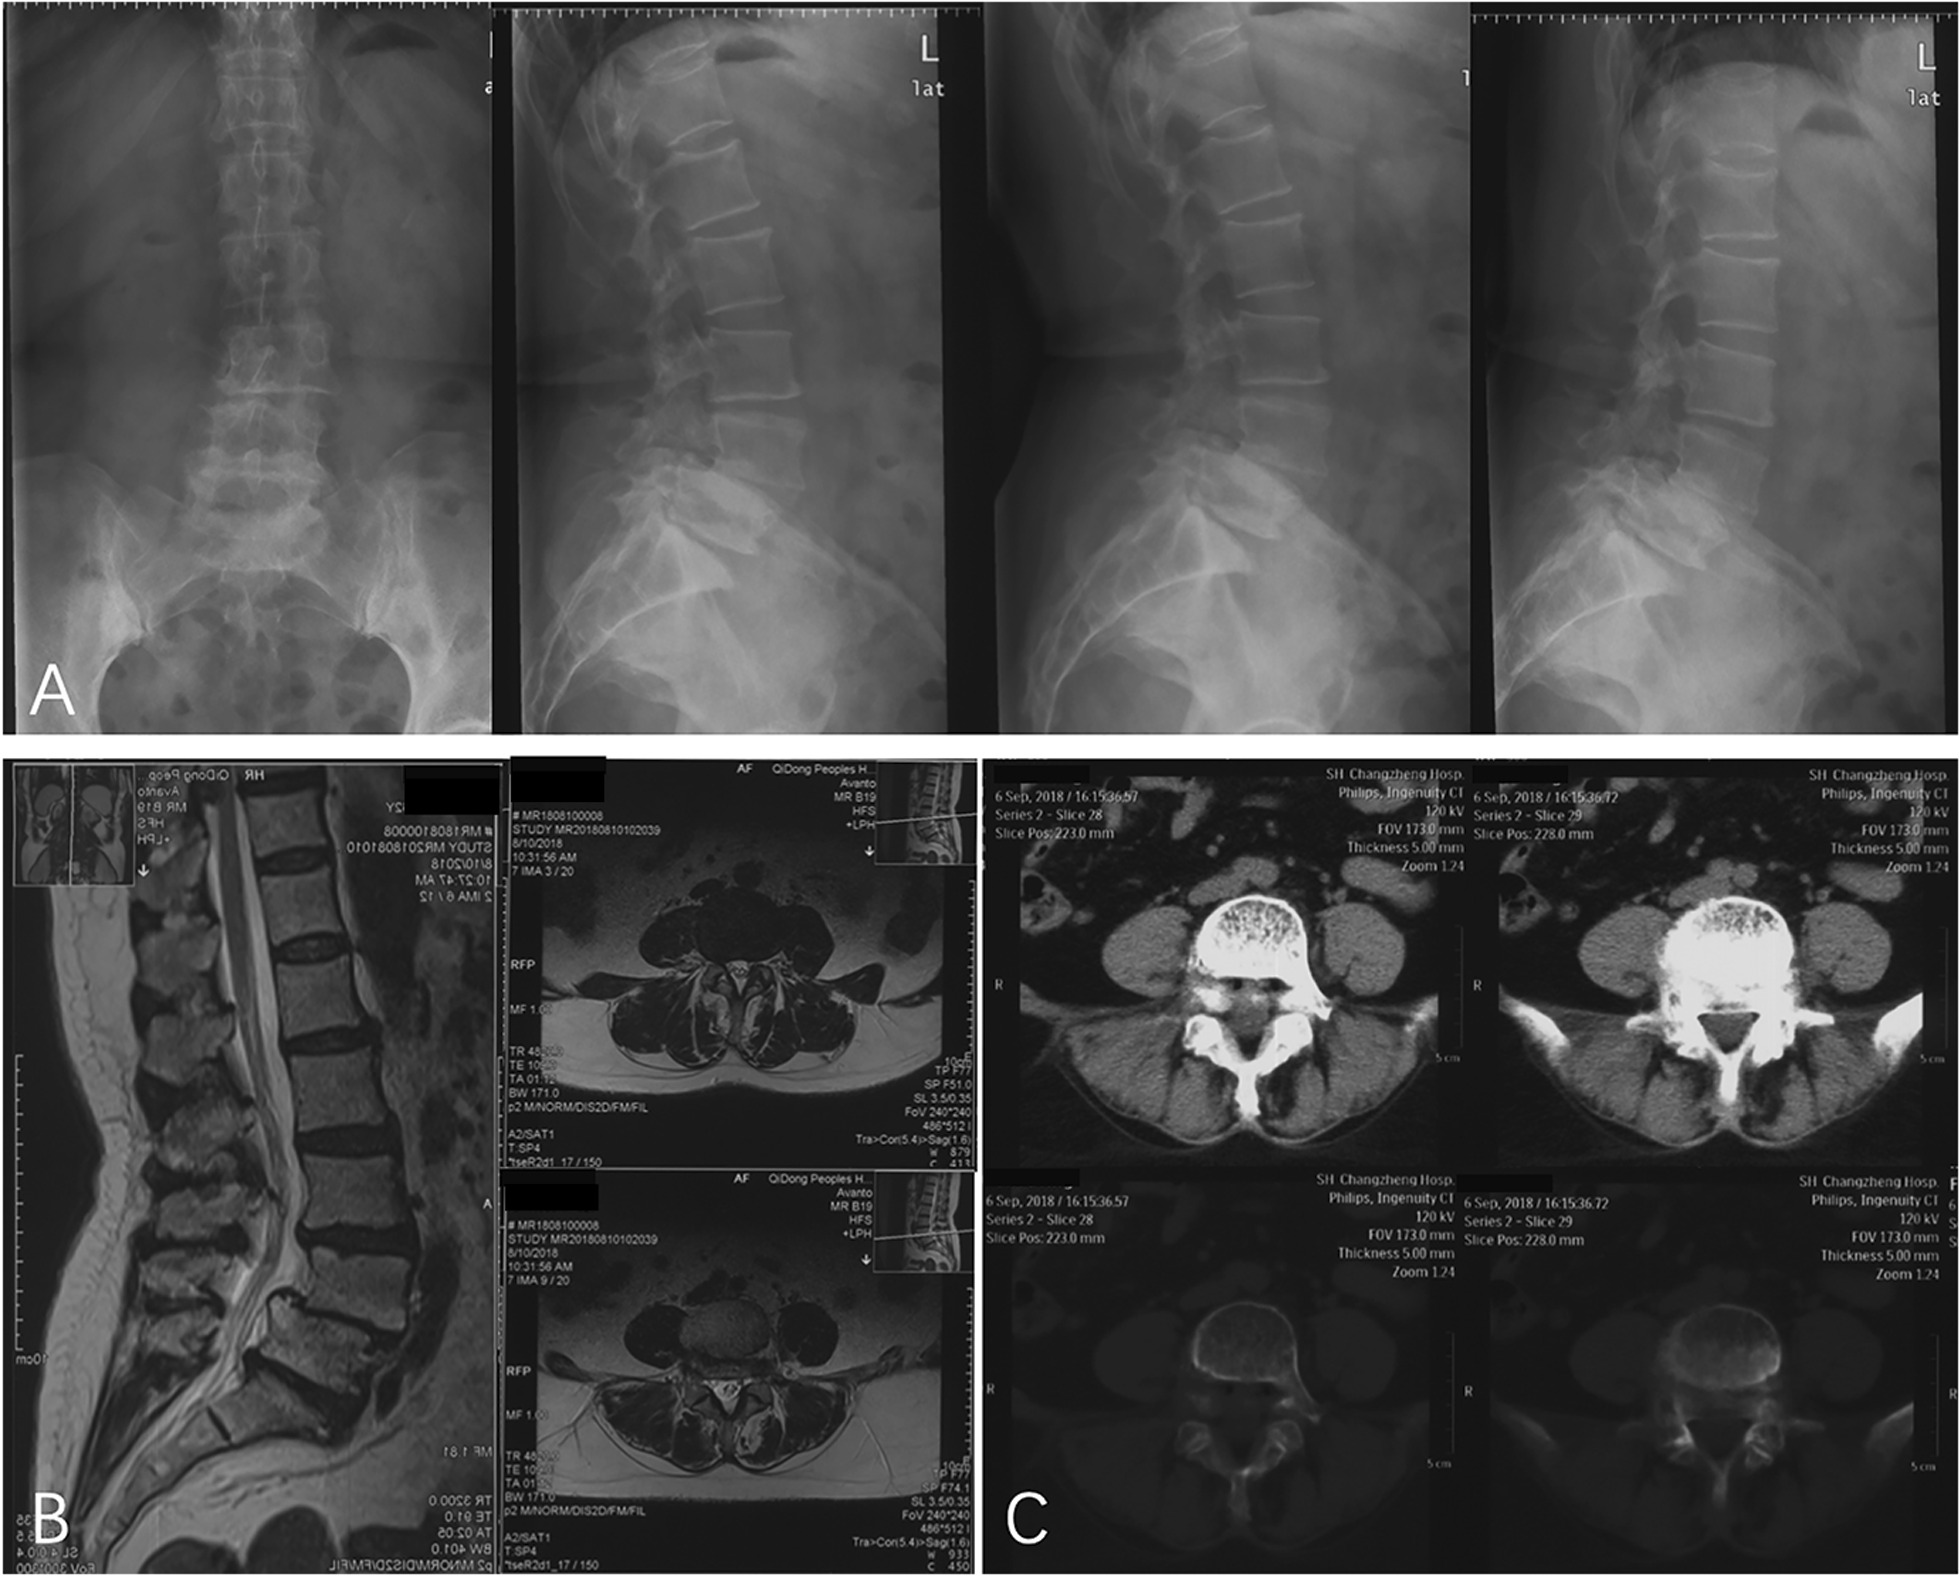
Preoperative radiography lumbar vertebrae, lateral position, overextension (a), MRI (b) and CT (c) revealed L4 vertebral body slip forward (I degree) and L4/5 lumbar spondylolisthesis
